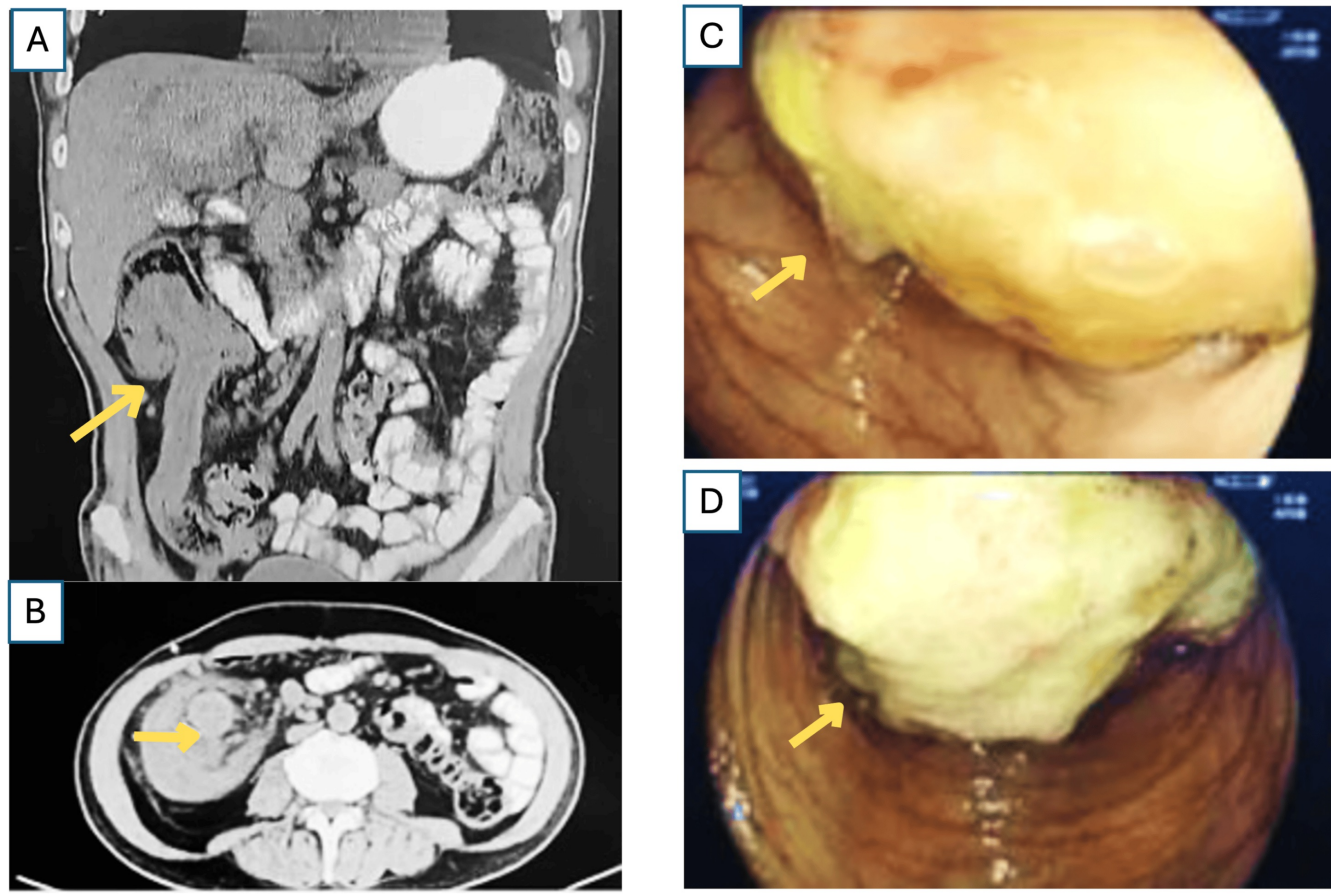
Diagnostic imaging of adult intussusception 1A (coronal) and 1B (axial) Abdominal computed tomography (CT) scan: The axial view of the abdominal CT scan shows the classic "target sign" of an intussusception (indicated by the arrow) in the right colon. The image clearly demonstrates a soft-tissue mass at the center of the invagination, acting as the pathological lead point. Note the presence of surrounding edema and mesenteric fat, which are also consistent with the diagnosis. 1C and 1D Colonoscopy findings: Endoscopic view from the colonoscopy showing a large, fungating tumor with irregular edges (indicated by the arrow) located in the ascending colon. The mass occludes approximately 90% of the lumen, which was identified as the lead point for the intussusception seen on the CT scan.
